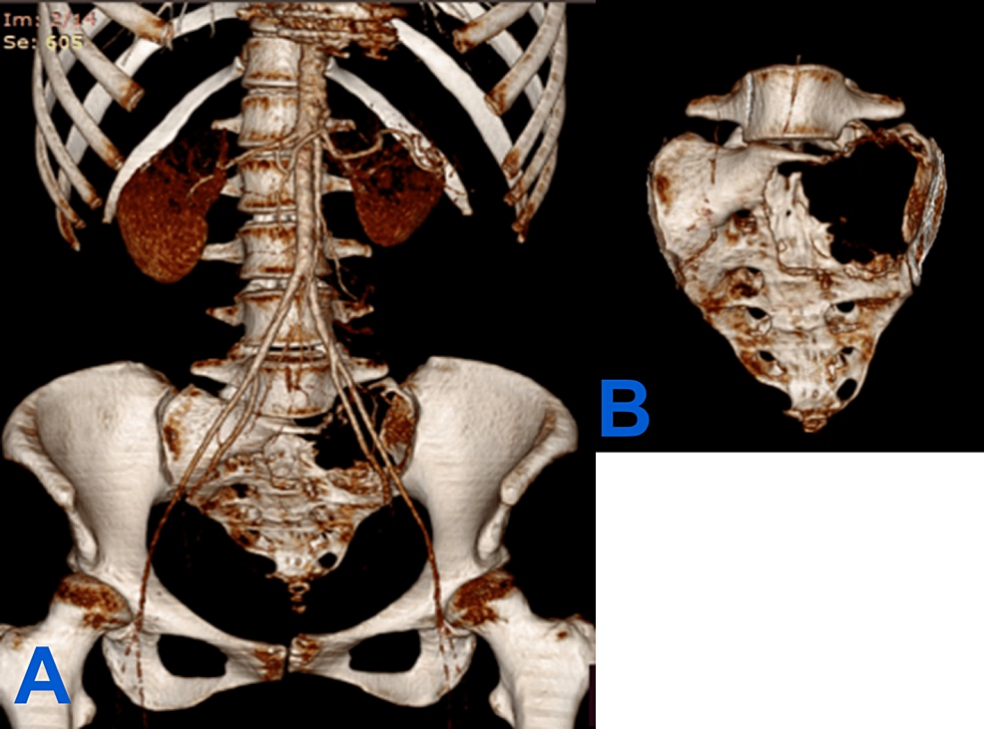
Preoperative planning with a 3D reconstruction of the CT. (A) Anterior view of lumbo-pelvic region with clear depiction of neighboring vital structures such as the internal and external iliac arteries along with what remains of the left sacro-iliac joint. (B) Anterior view of the sacrum showing the extent of the lesion allowing for correct planning of resection amount.
